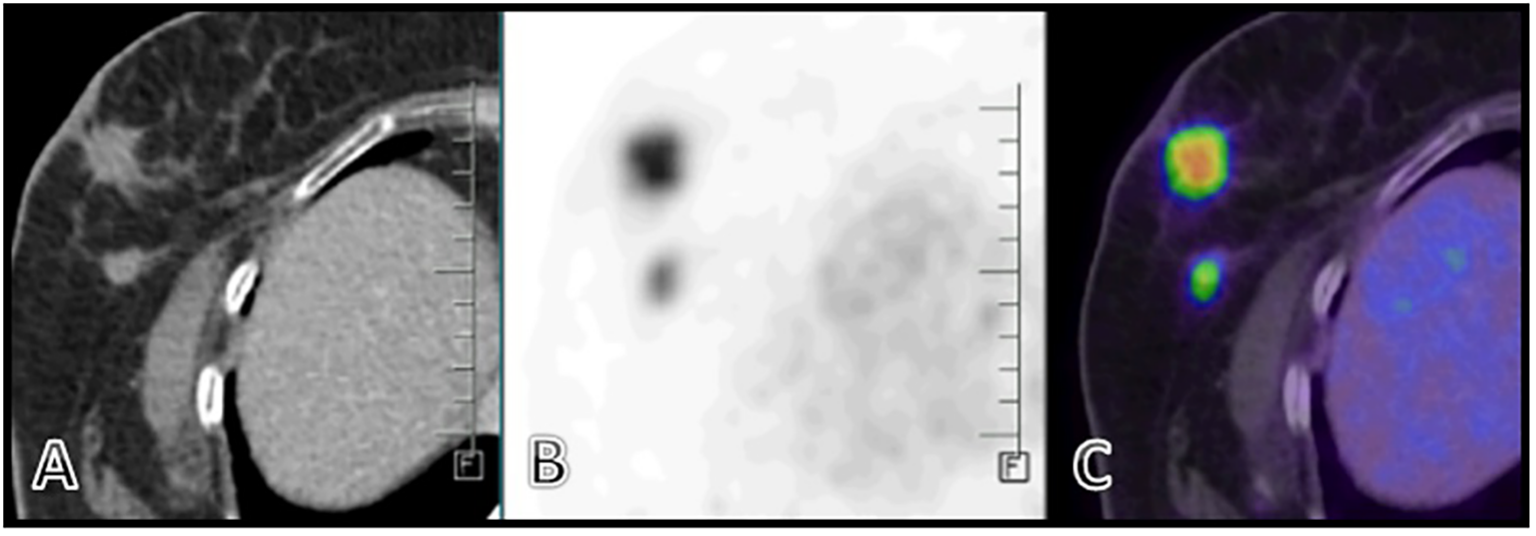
(A–C) Ill-defined spiculated FDG avid (SUV max 10.8) mass lesion seen in the outer quadrant of the right breast measuring 22 x 21 x 21 mm,. A few thin fibrous strands seen extending from the lesion to the overlying mildly thickened skin (2.5mm). Two other FDG avid nodules were also seen.
